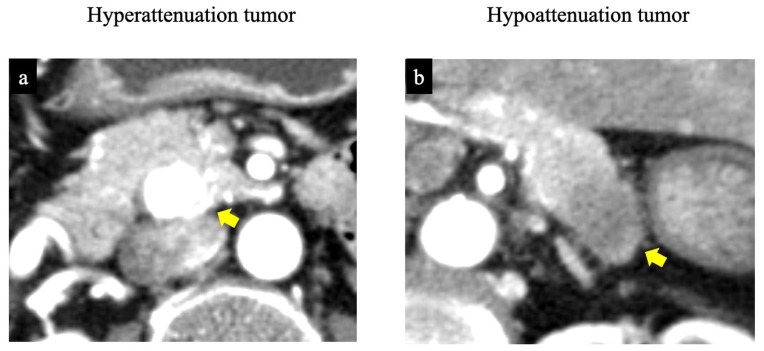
Findings of contrast-enhanced computed tomography: (a) Hyperattenuated tumor (arrow) showing higher tumor attenuation than the pancreatic parenchyma (Hounsfield unit ratio of tumor to pancreatic parenchyma during the pancreatic parenchymal phase ≥1). (b) Hypoattenuated tumor (arrow) showing lower attenuation than the pancreatic parenchyma (Hounsfield unit ratio during the pancreatic phase <1).
